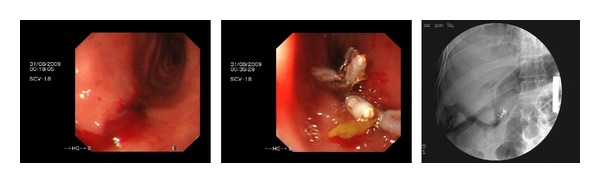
The duodenal lateral perforation was sutured by clips. The gas in peritoneal cavity was shown by X-ray.
